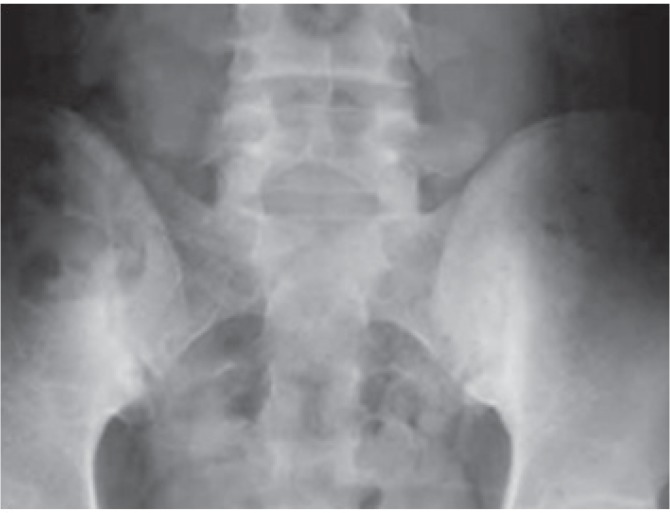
Sacroiliac joint radiography demonstrating bilateral sacroiliitis
